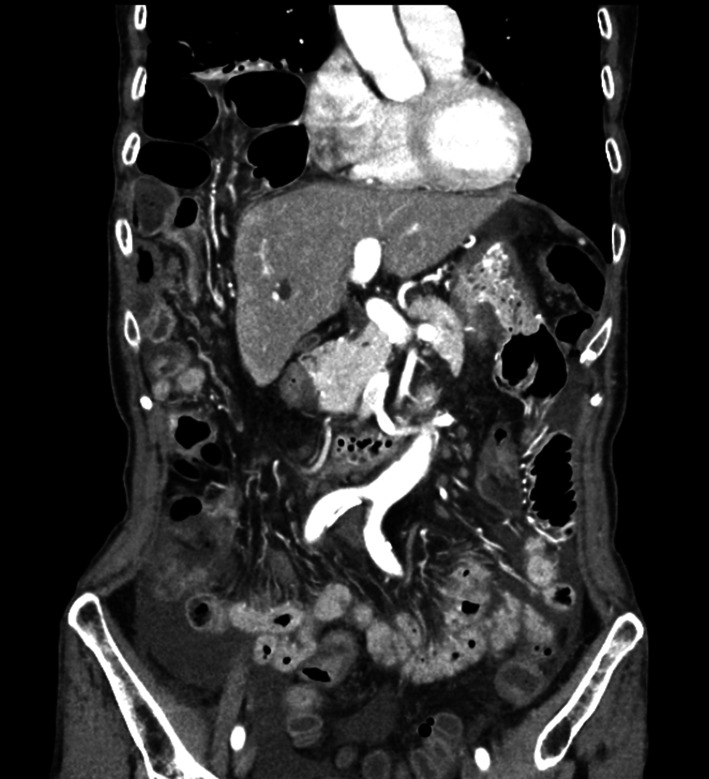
Contrast-enhanced computed tomography demonstrating herniation of the small intestine into the thoracic cavity
